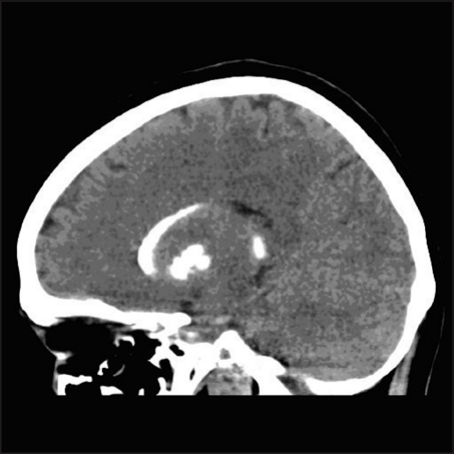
CT scan of the brain: Sagittal plane shows calcifications of the thalamus and corpus striatum.
